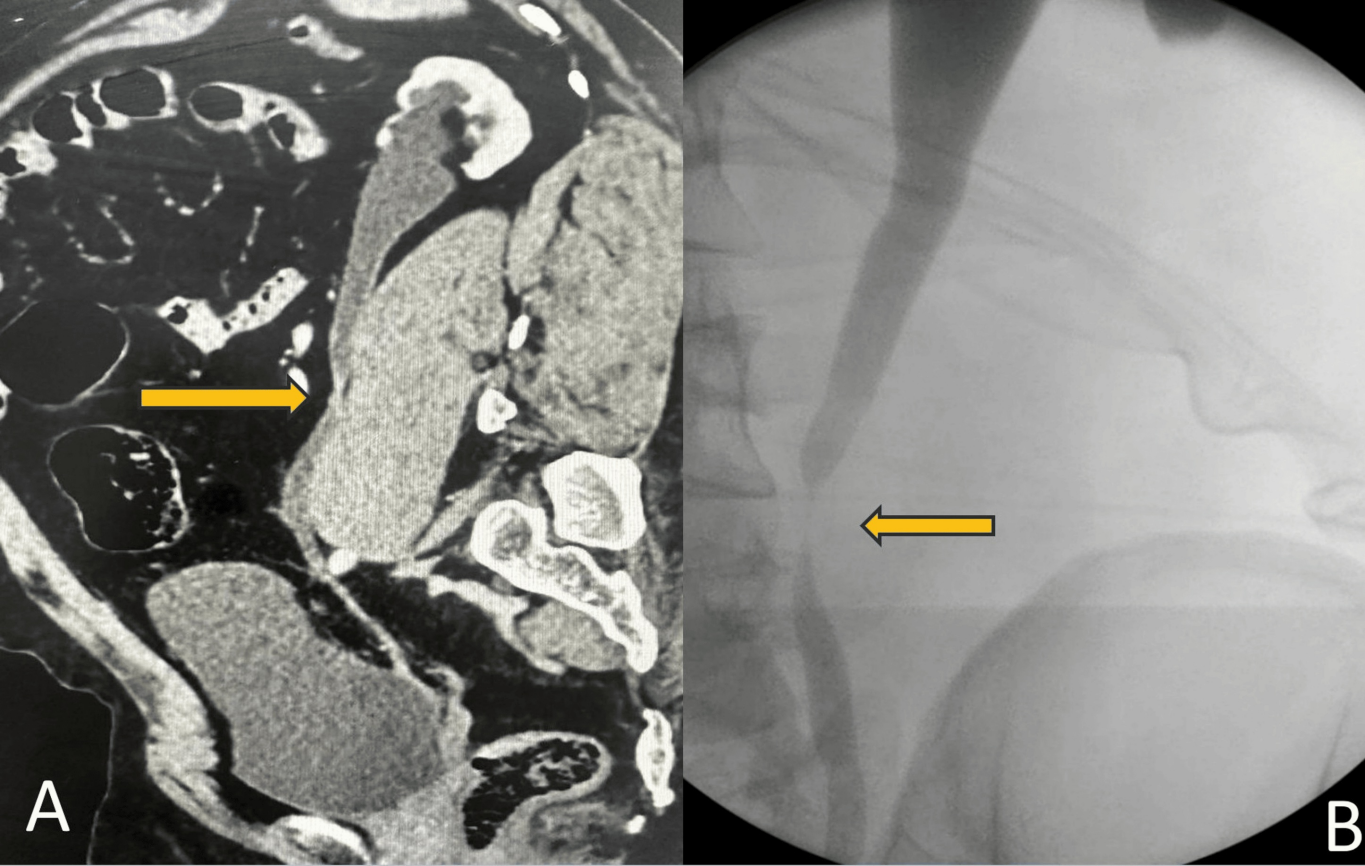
(A) Computed tomography image showing upper ureteric stricture in sagittal view; (B) retrograde pyelogram confirming the stricture site.
